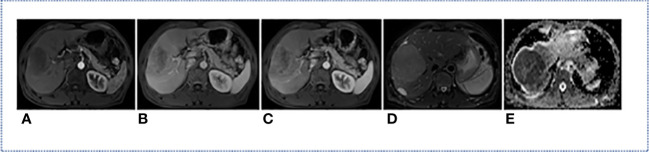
MRI modality of one HCC Patient. (A) Arterial Phase; (B) Delay Phase; (C) Venous Phase; (D) T2-Weighted; (E) apparent diffusion coefficient.
